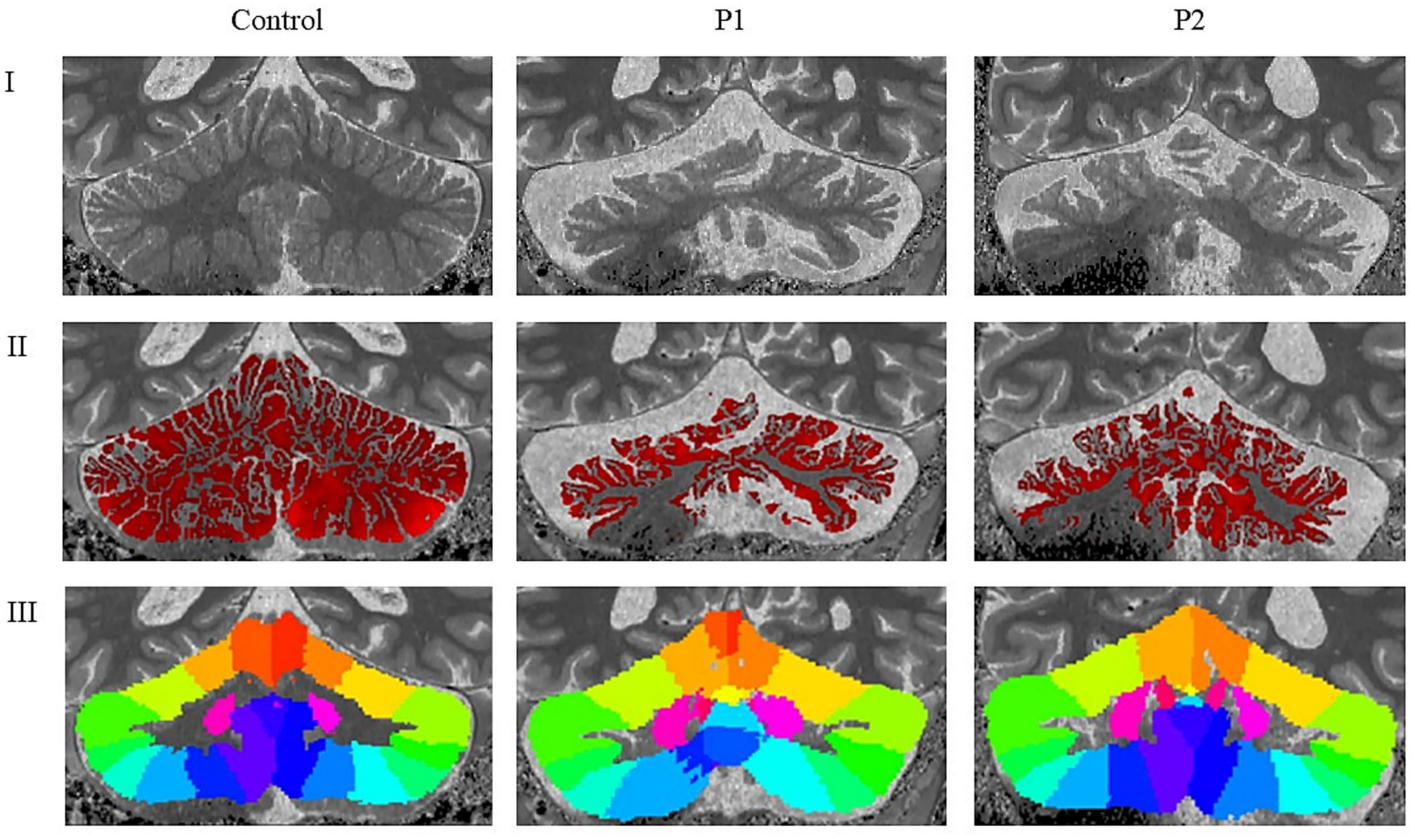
Neuroradiological findings in healthy control, P1 and P2. I: Cropped and rescaled T1-weighted anatomical image; II: Cerebellar cortical thickness map derived from the T1-weighted image; III: Cerebellar lobular atlas warped to the subject’s native anatomical space; P1 = Individual 1 with SCA34; P2 = Individual 2 with SCA34.
